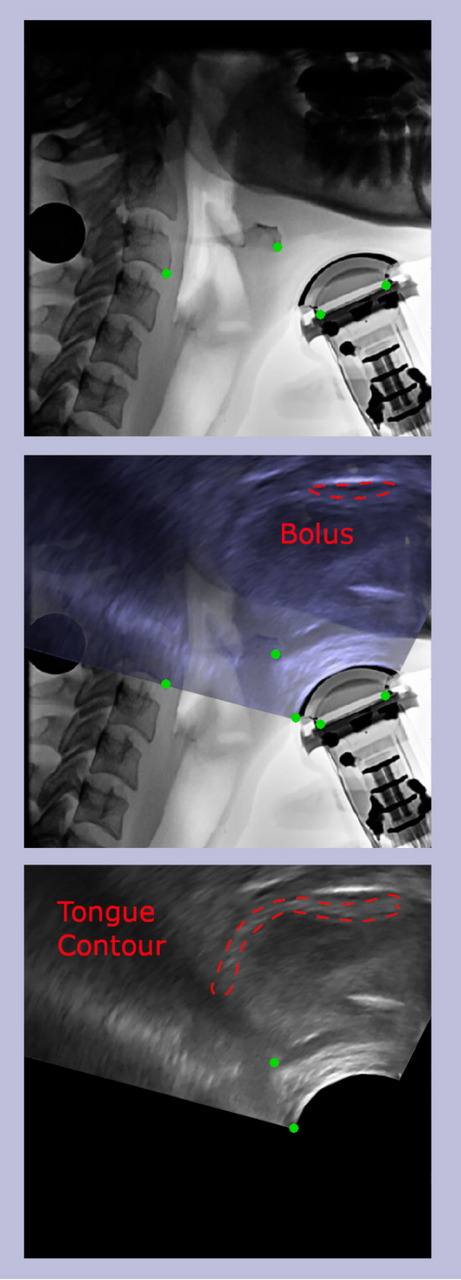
Representative synchronous ultrasound and videofluoroscopic (MBSS) imaging. Top: MBSS image frame. Bottom: Labeled ultrasound B-mode frame after registration to MBSS. Middle: Co-registered, blue-tinted B-mode ultrasound frame overlaid onto MBSS frame. Green dots in each panel correspond with tracked landmarks
